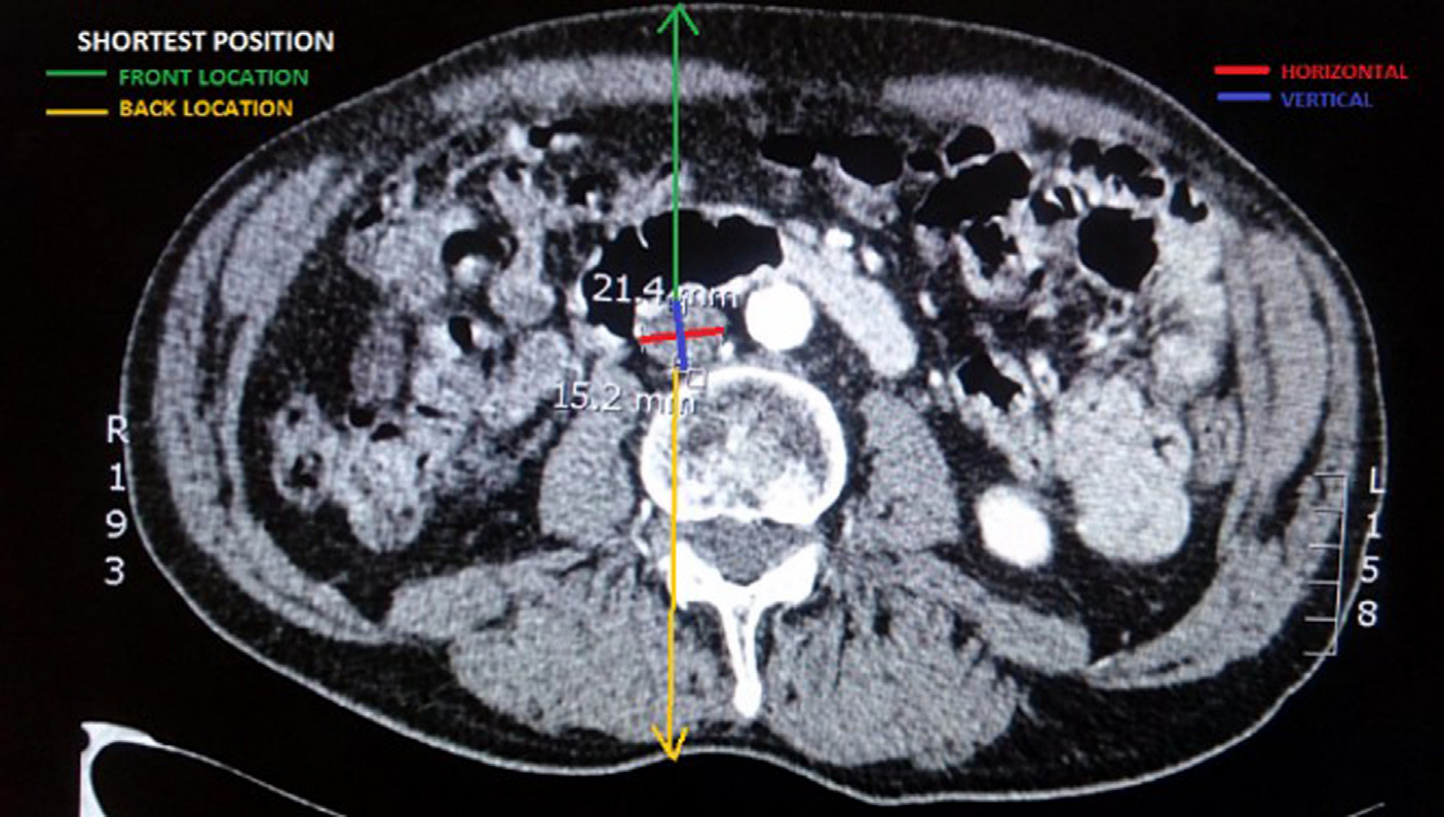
CT scan showing how measurements of diameter and link distances were taken. Link distance was measured both to the front and the back of the patient, as wireless collection of data can be done from either side of the patient.
